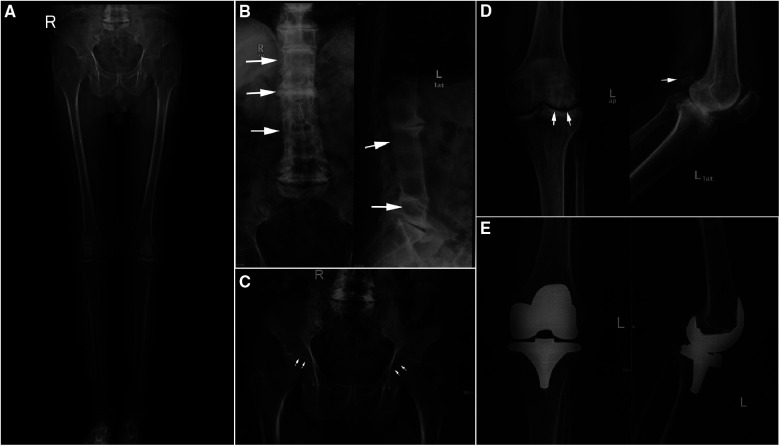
Pre and postoperative digital radiation (DR) image. (A) Preoperative full leg weight bearing x-Ray of both lower extremities. (B) Preoperative anteroposterior and lateral DR views of lumbar. (C) Preoperative anteroposterior DR view of pelvic. (D) Preoperative anteroposterior and lateral DR views of left knee. (E) Postoperative anteroposterior and lateral DR views of left knee.
